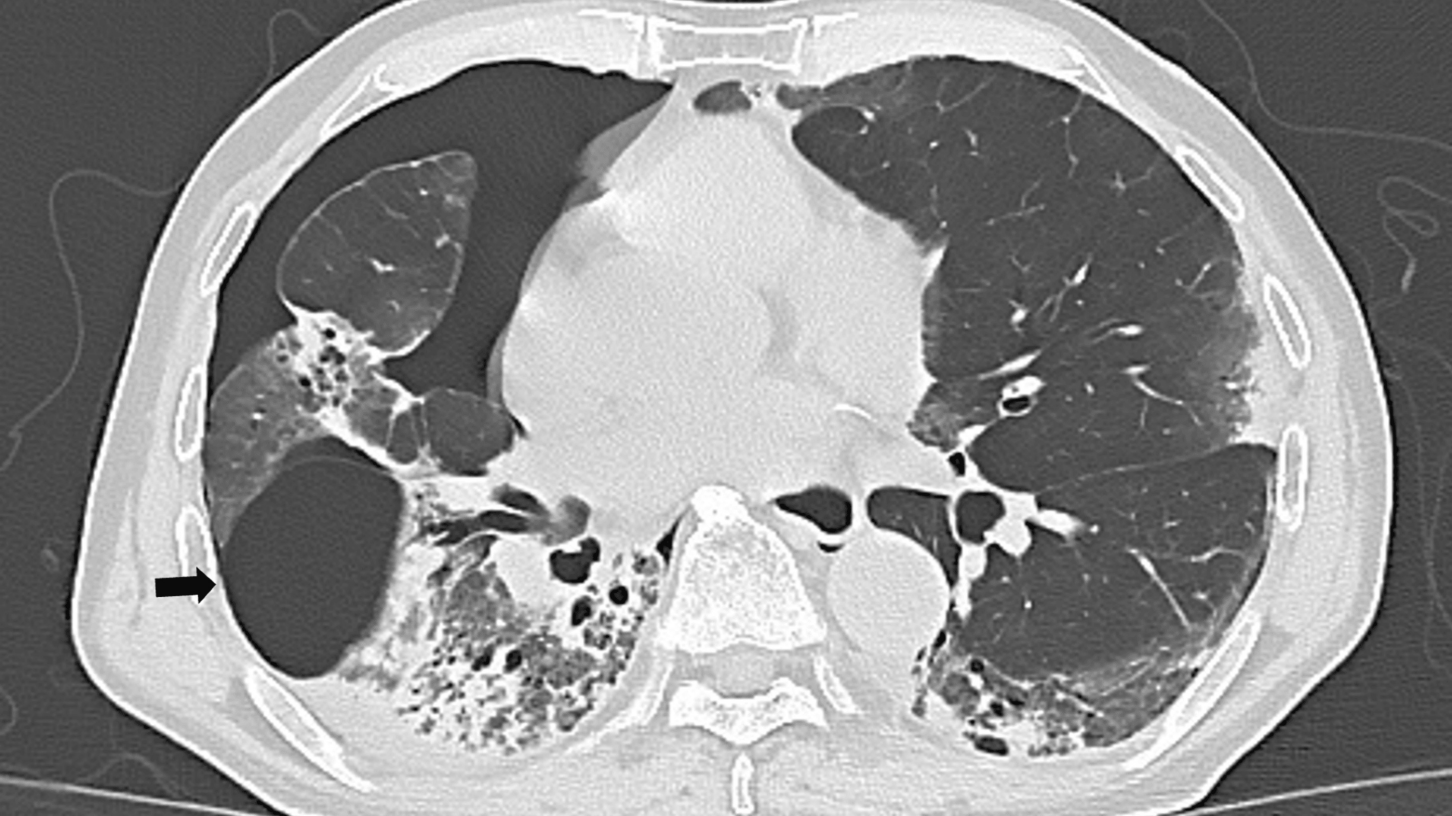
Chest CT on day five of readmission showing a right pneumothorax and a newly appeared 5-cm thin-walled, air-filled cavity in the right lower lobe, consistent with a pneumatocele (arrow).
